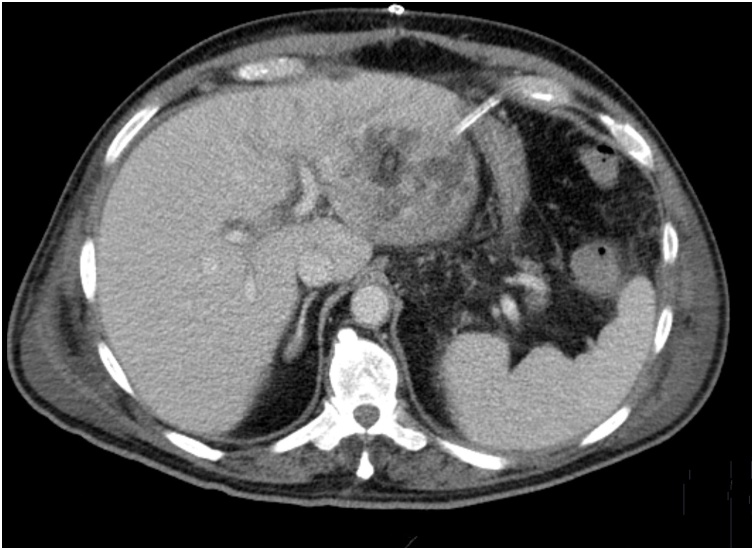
Abdominal CT scan seven days after surgical drainage. An ongoing multiloculated abscess in the left lobe is identified with a drain inside.
